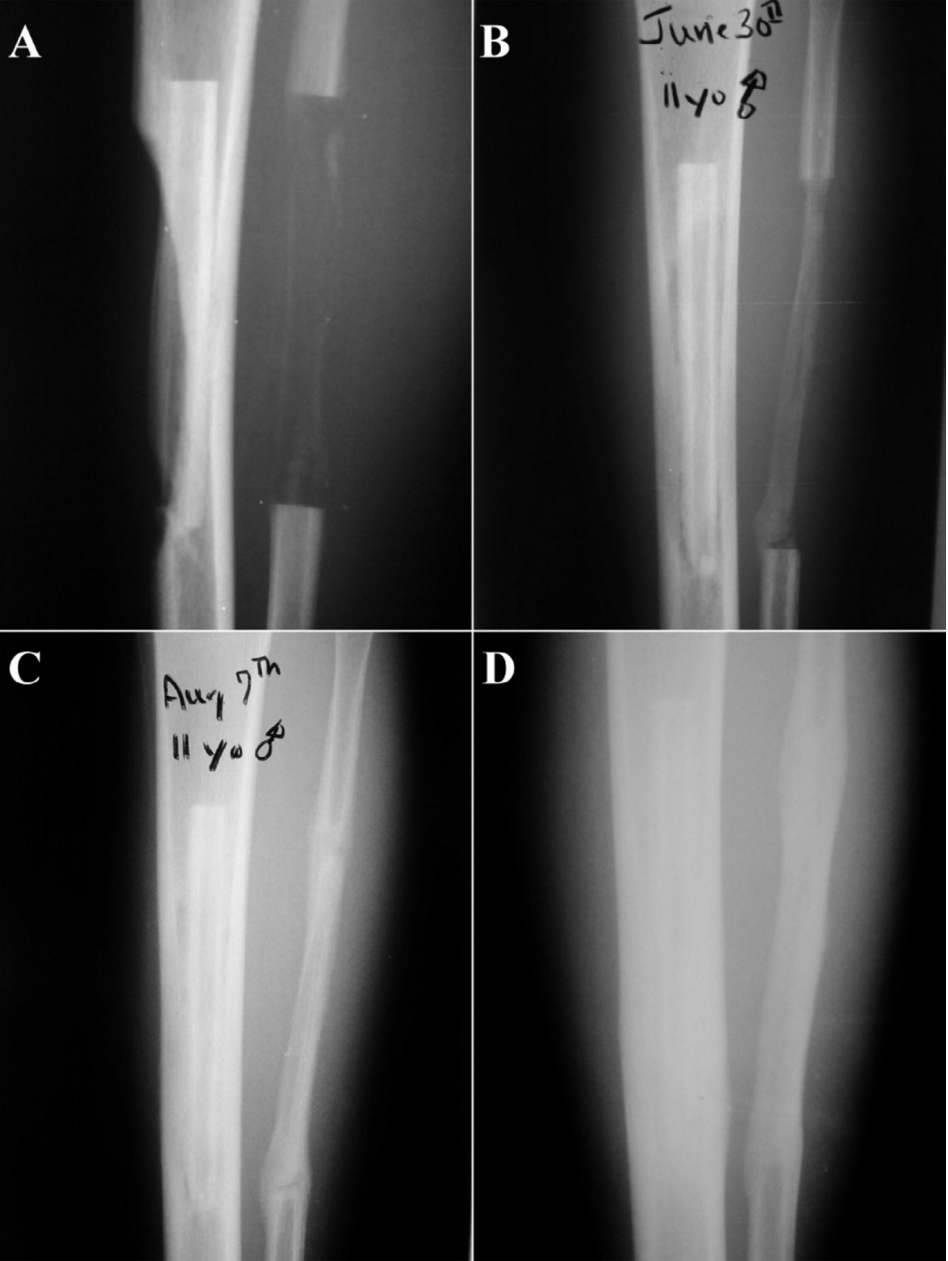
11 year male with malignant tumor. Fibula-pro-tibia following local resection. Cortical regeneration from periosteum. Performed at Mt. Sinai Medical Center, NYC, 2000. A: 3 weeks post-operative radiograph, B: 6 weeks post-operative, C: 3 months post-operative, D: approximately 6 months post-operative.
